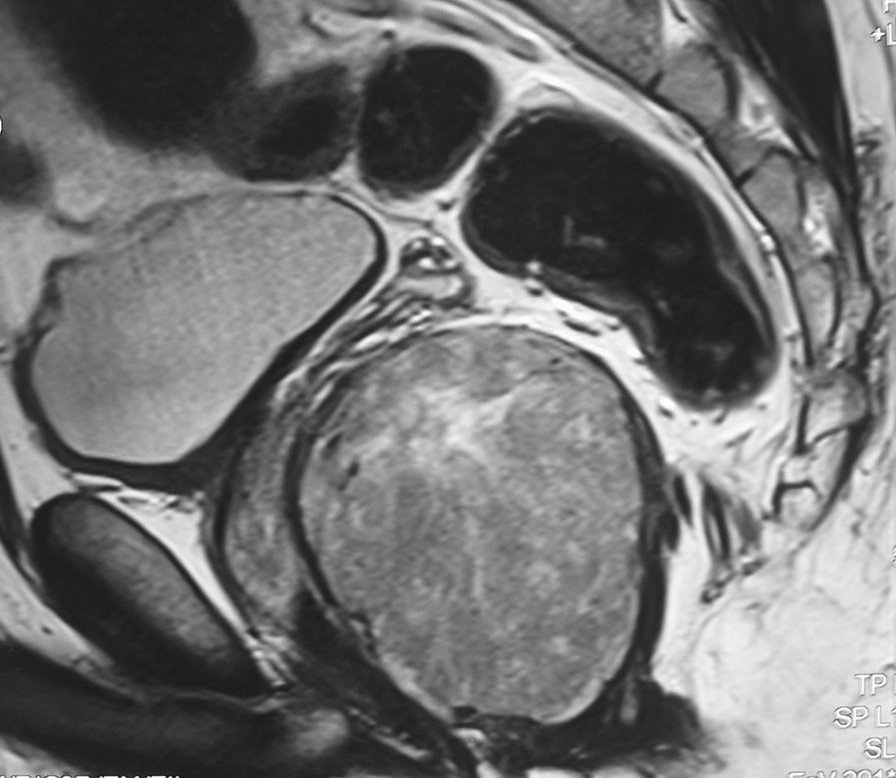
Magnetic resonance imaging findings: sagittal T2-weighted image showing a large pelvic mass with high signal intensity and heterogeneous content but without bone or bladder invasion or any evident communication with the lumen of the rectum
